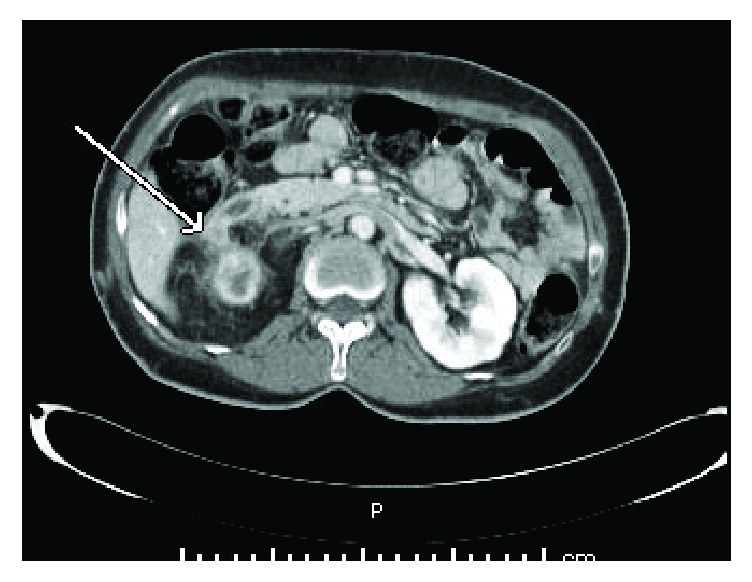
CT scan of the abdomen demonstrating a fistula between the upper pole of the right kidney and the duodenum.
